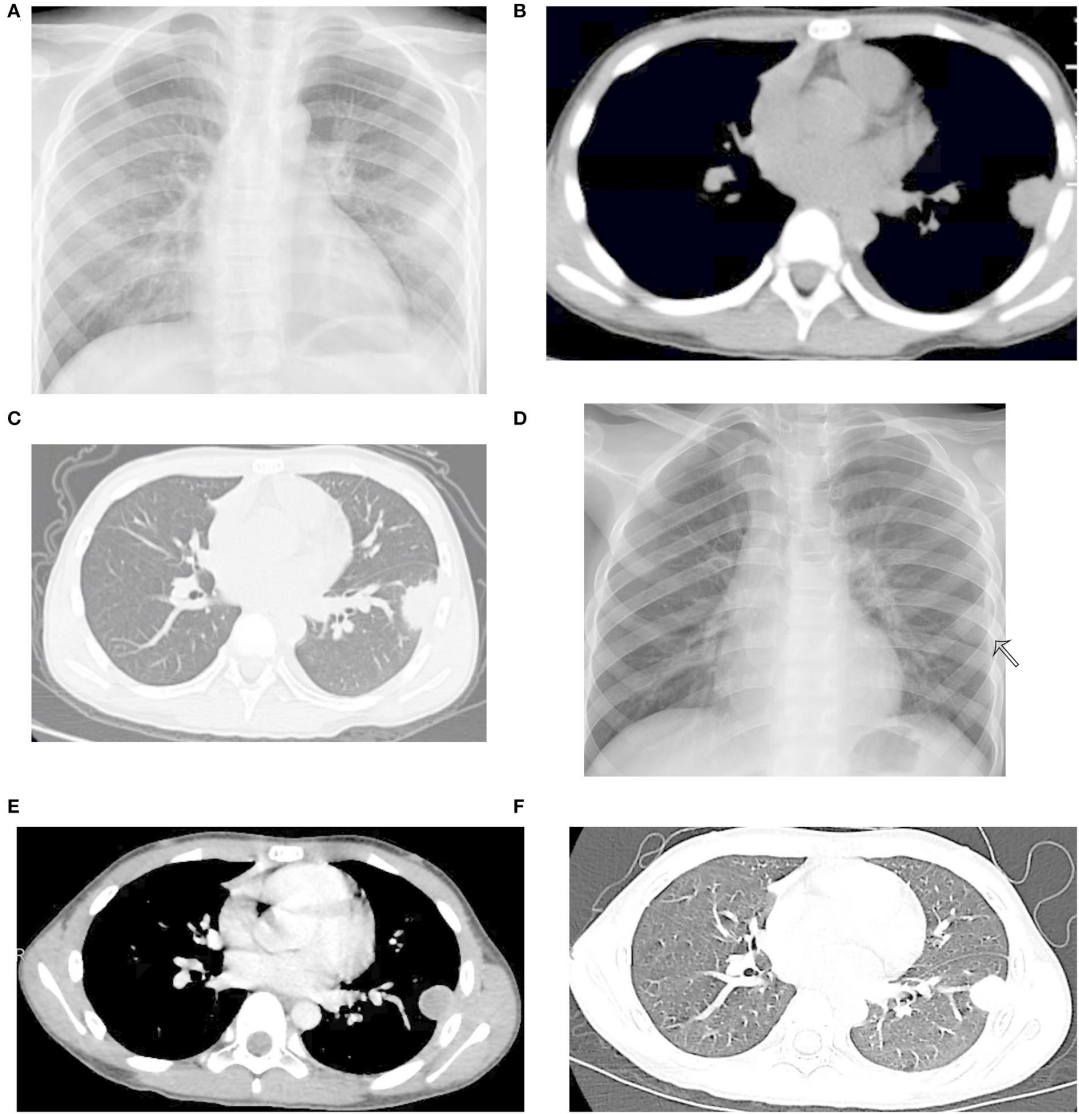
Radiological images before ultransonography (US)-guided lung biopsy. (A) Signs of infiltration present in the left lower lung field. (B,C) Plane computed tomography (CT) at the previous hospital revealing a round soft tissue mass and a small amount of pleural effusion in the left lung. (D) Chest X-ray revealed a left lung nodule measuring 2 cm in diameter. (E,F) Contrast-enhanced CT of the chest showing a rim-enhancing lesion in the left lower lobe lung.
